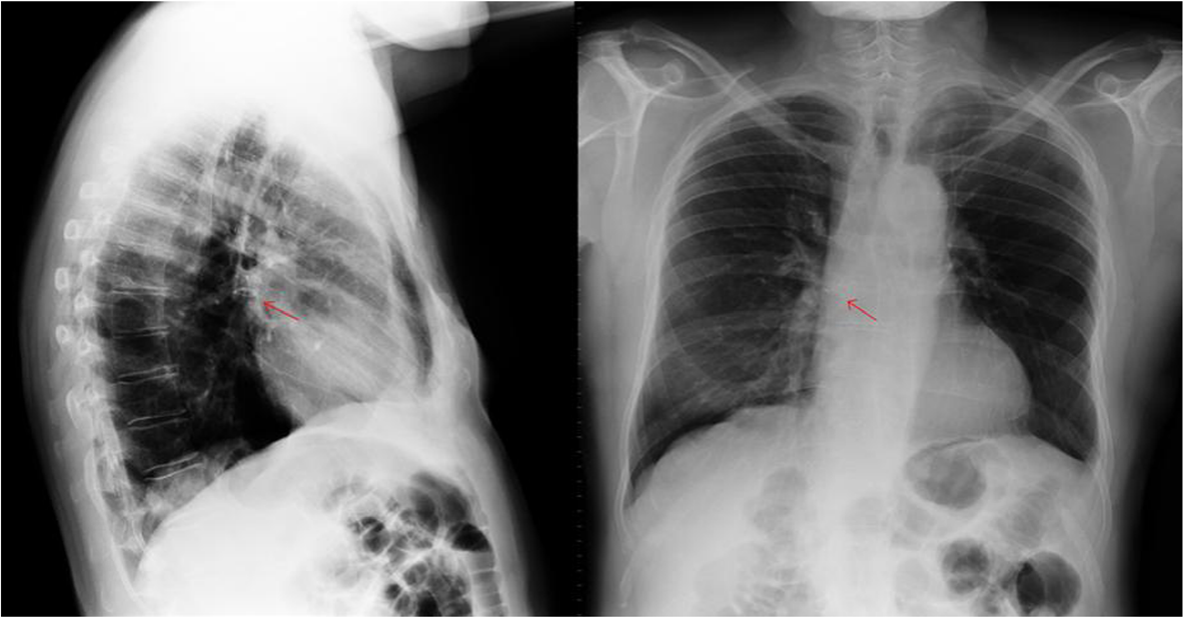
Chest radiograph showing a ring-shaped opacity in the right mainstem.
